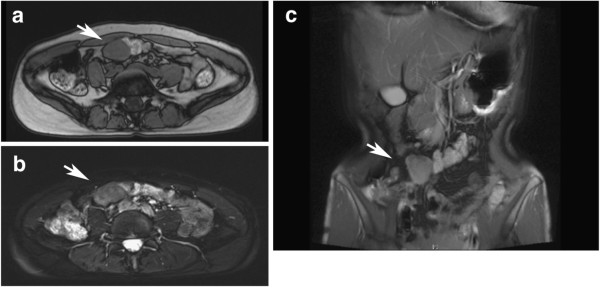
Magnetic resonance imaging findings showed the desmoid tumor (arrows) with lower signal intensity on T1-weighted image (a) and high signal intensity on T2-weighted image (b and c). Note that the desmoid tumor was next to contrast-filled transverse colon. (a and b) Axial plane. (c) Coronal plane.
